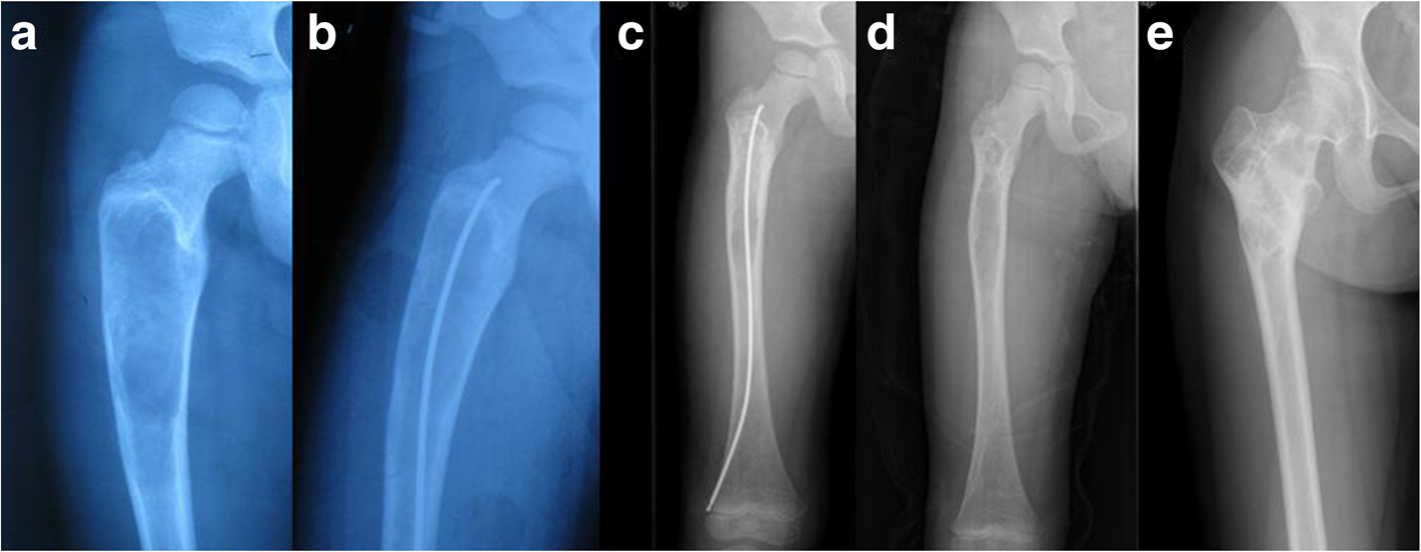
Radiographs of a 5-year-old boy who presented with leg pain and loss of function. a A bone cyst is present in the proximal metaphysis of the femur without pathological fracture. b Flexible intramedullary nailing was used to fix and decompress the bone cyst. c The lesion shows signs of healing 6 months after surgery. d At 26 months the bone cyst is healed and the nail is removed. e The lesion is completely healed at 59-month follow-up
